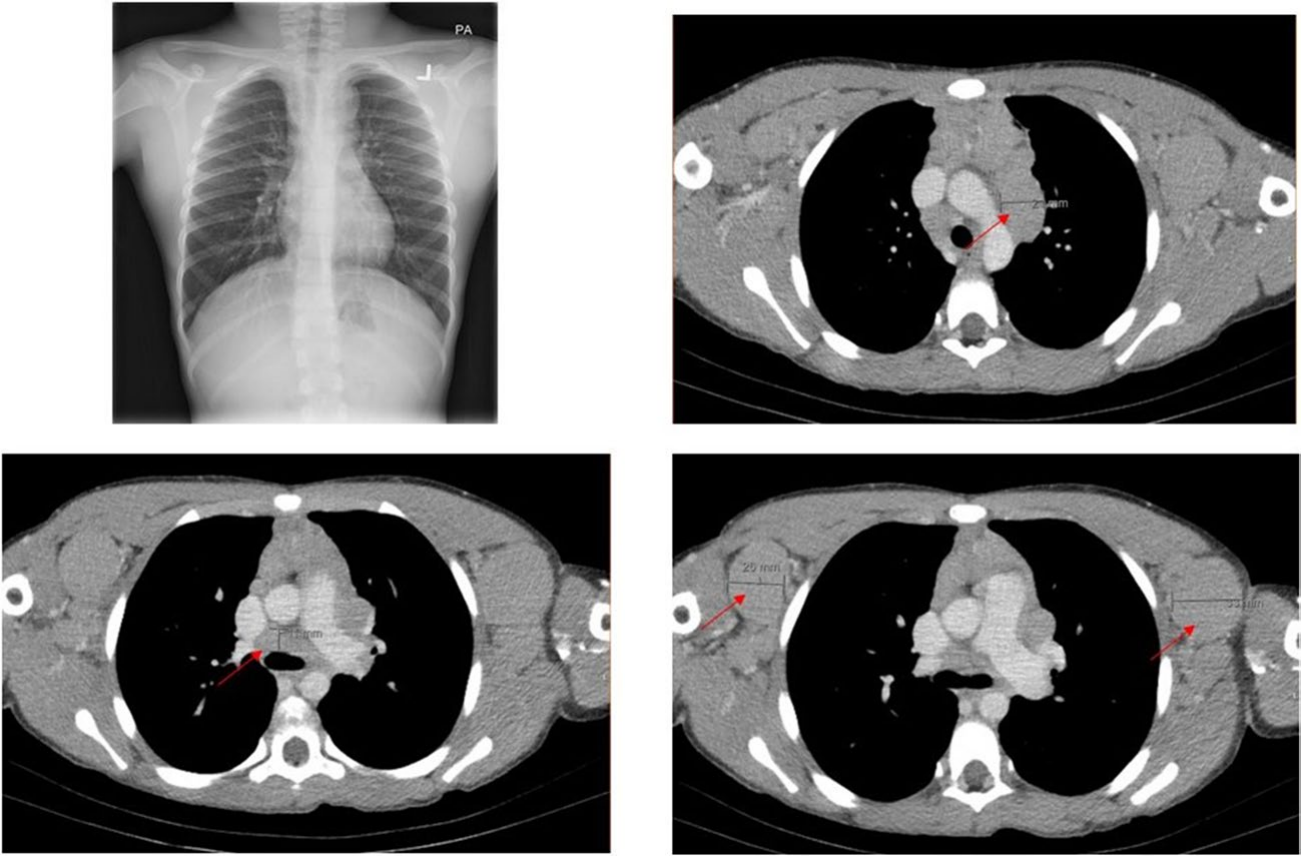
A Chest X-ray demonstrating right paratracheal stripe, suggesting mediastinal lymphadenopathy. Subsequent CT imaging of the chest with IV contrast demonstrated multiple enlarged lymph nodes within the B, C mediastinum and D bilateral axillae
